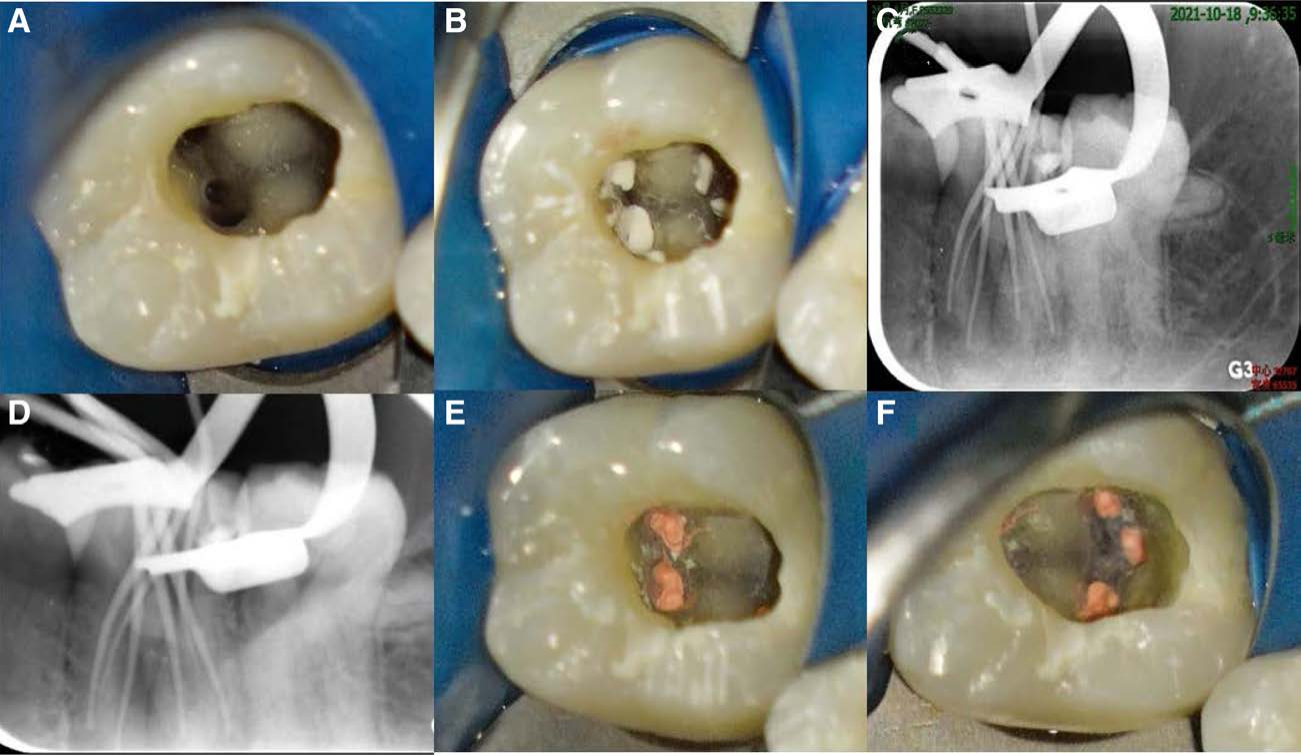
Root canal filling procedure of tooth 36. (A–B) Cleaning and filling the root canals with iRoot SP root canal sealer. (C–D) Periapical radiographs of gutta-percha cones in root canals. (E–F) Intraoral photographs of pulp chamber after root canal filling.
